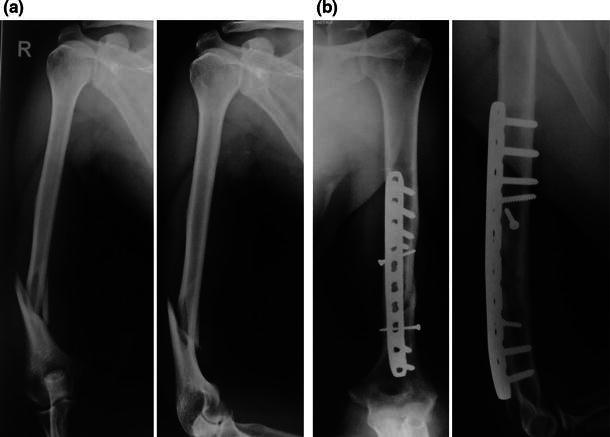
a Anteroposterior and lateral views showing an oblique fracture with medial butterfly fragment. b Immediate post-operative radiographs showing fixation with two lag screws and pre-contoured LCP with two locking screws in the distal fragment
